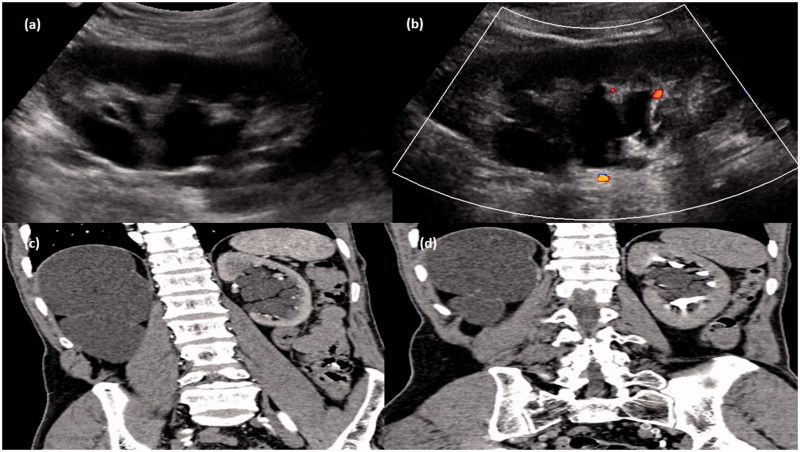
Gray-scale ultrasound image (a) of left kidney showing an echoiec collection in peripelvic region which showed no evidence of color flow (b) on color Doppler study. CT scan arterial phase (c) and delayed phase (d) in coronal plane showing collection in peripelvic region with no evidence of contrast within it. The grossly hydronephrotic contralateral kidney is also seen.
